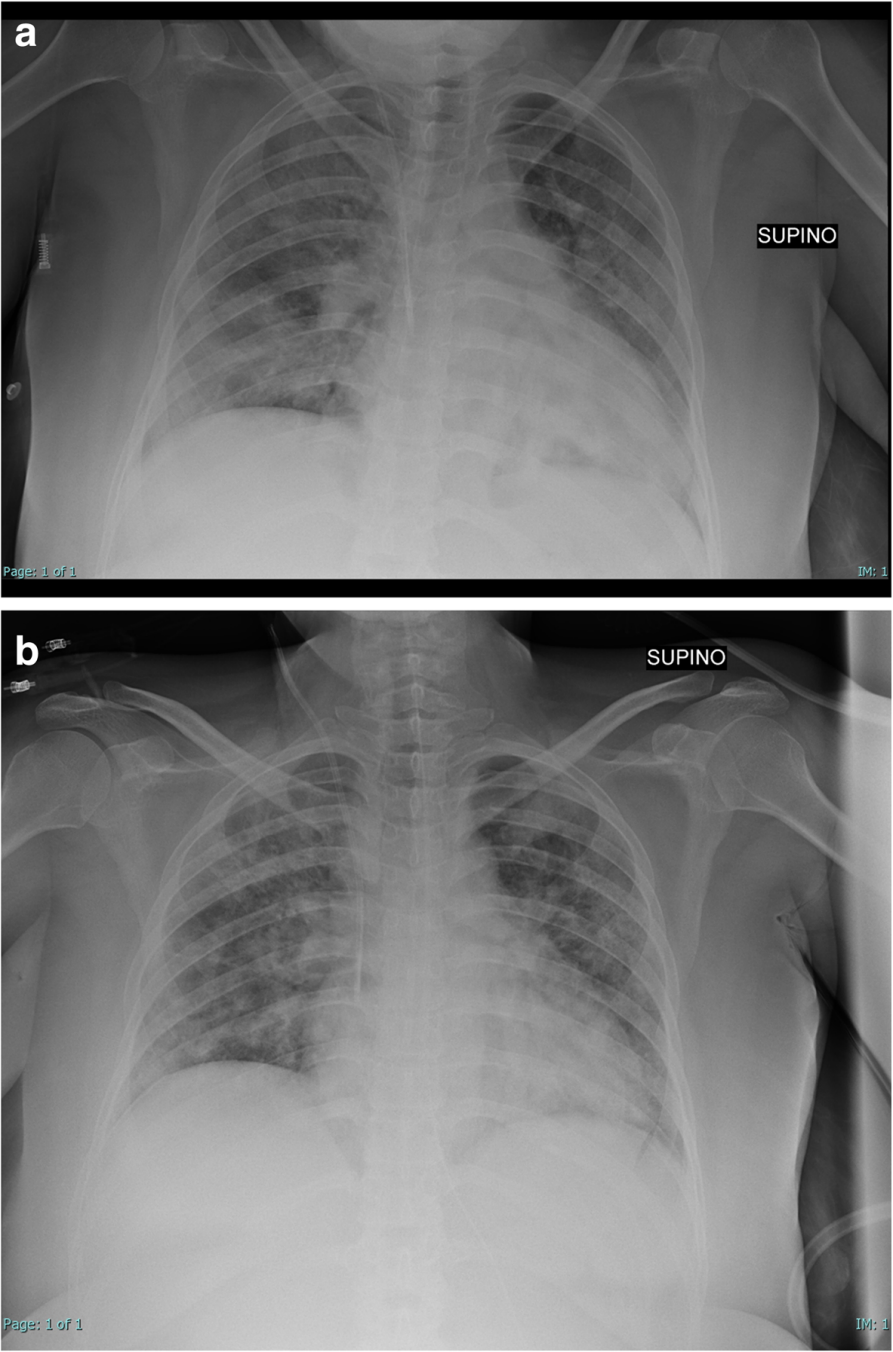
a Chest radiography of the pregnant woman before intubation, at ICU admission. b Chest radiography of the pregnant woman after extubation (15th day)
